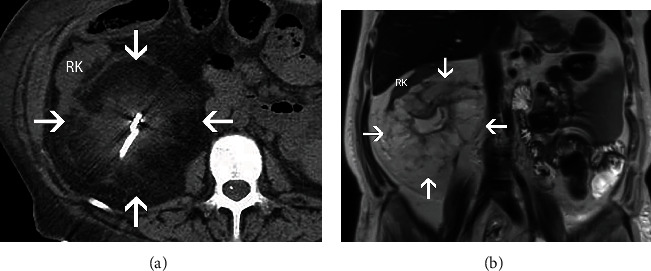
Imaging studies of the right kidney. (a) CT of the abdomen without contrast, axial view. At the level of the mass (outlined by white arrows), the right kidney (RK) is atrophic and there is extensive lipomatous proliferation of the renal sinus fat. The bright linear structure in the middle of the mass is a percutaneous drainage tube. (b) MRI of the abdomen without fat suppression or contrast, coronal T2- weighted image. There is a prominent hyperintense fatty proliferation of the renal sinus fat (outlined by white arrows) displacing the right kidney (RK). There is an associated thin rim of enhancing adjacent renal parenchyma.
